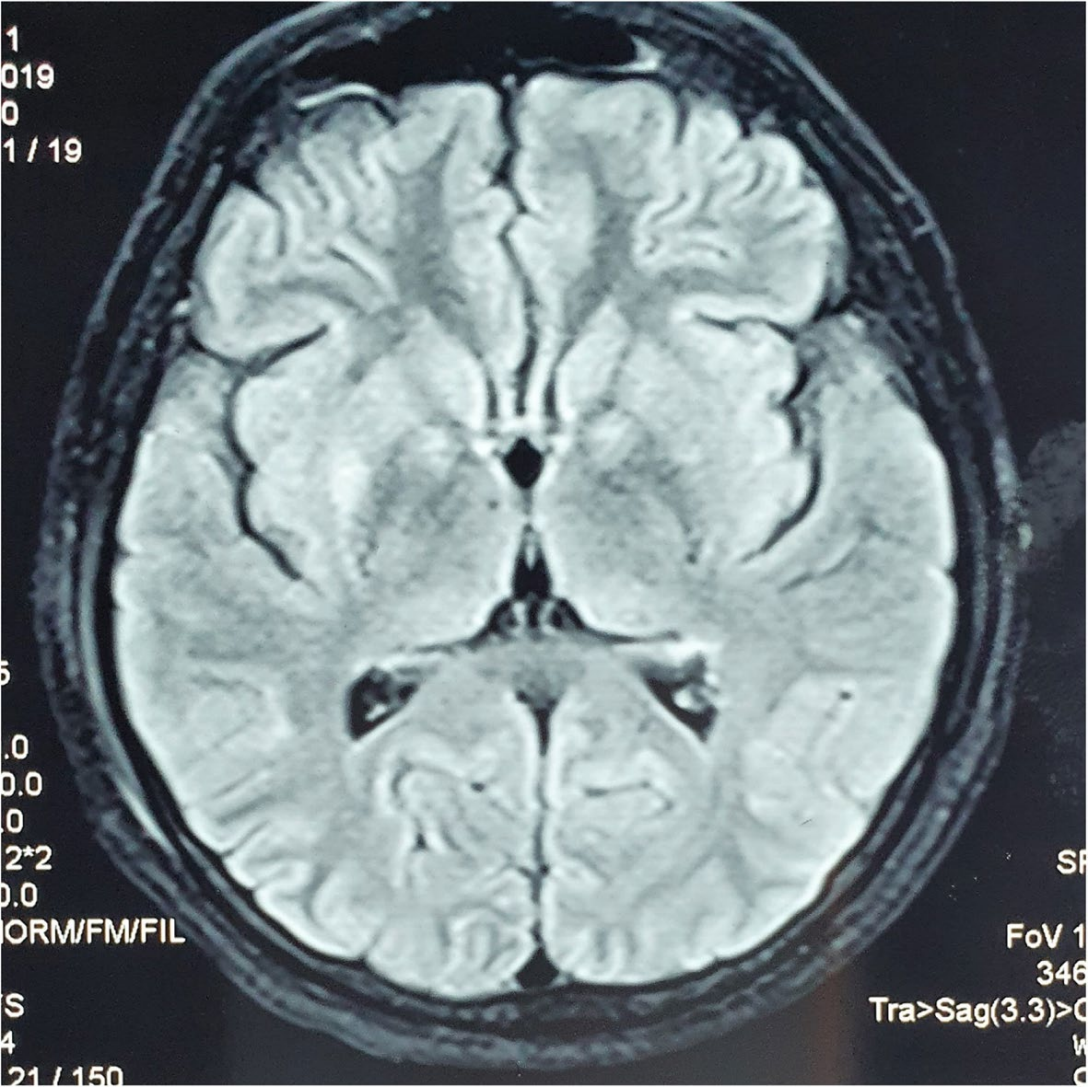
MRI Brain T2 FLAIR- Hypoglycaemic encephalopathy
